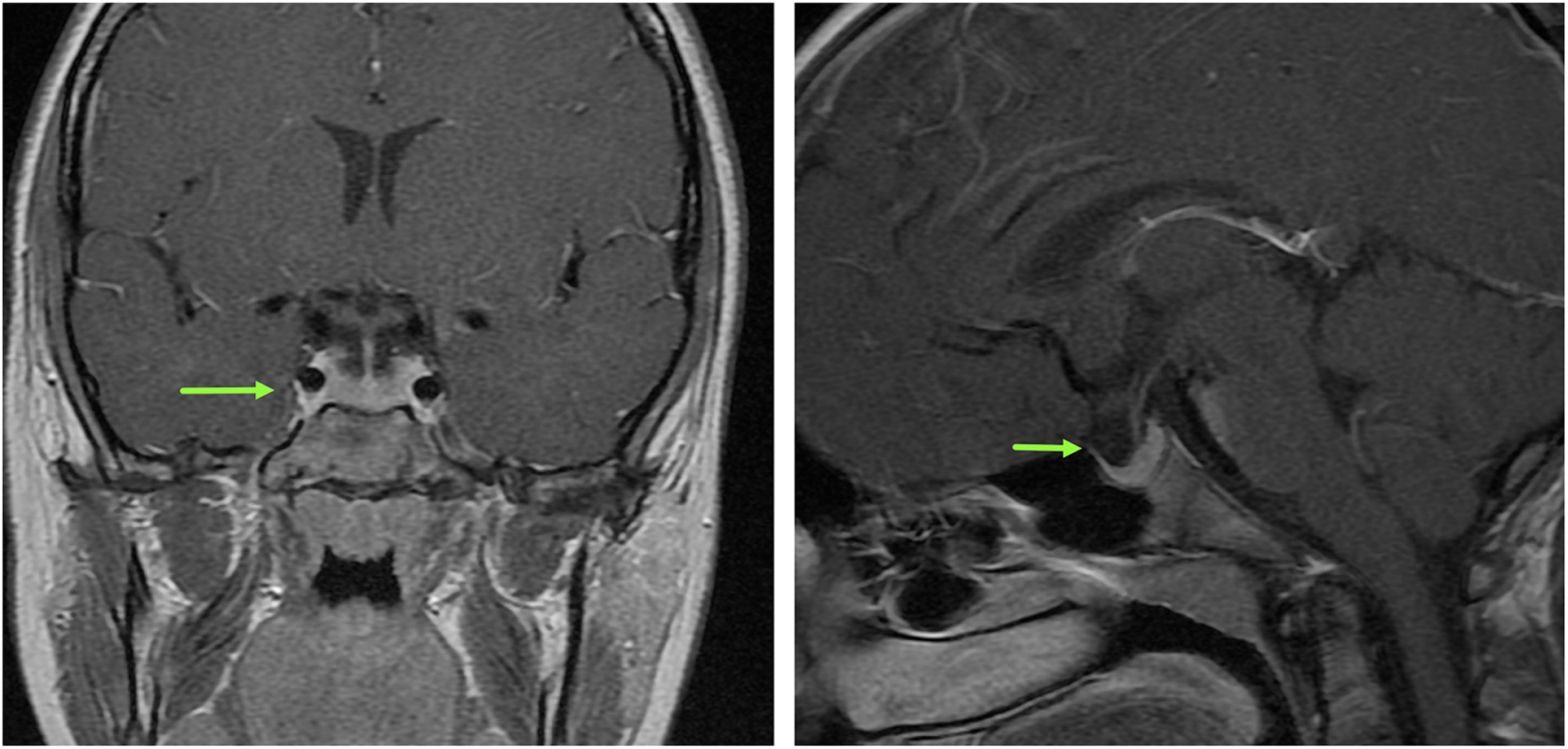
Normal pituitary on follow-up magnetic resonance imaging scan. Eighteen months after treatment with levothyroxine was initiated there was persistence of the enlarged sella (green arrows, coronal and sagittal views) with a normal positioned and sized pituitary gland including normal stalk and posterior bright spot (T1-weighted post-gadolinium images).
